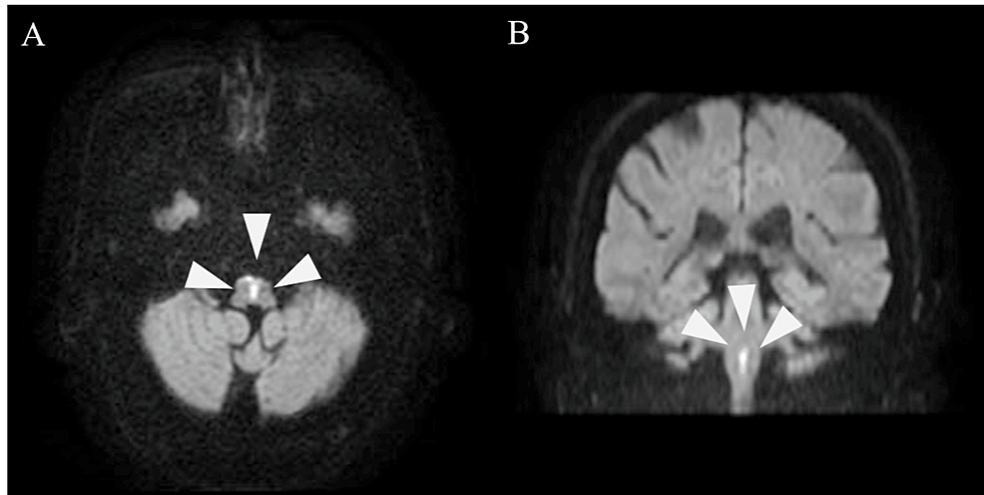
Head MRI findings on the day after admission. A: Axial DWI view. B: Coronal DWI view. High signal in the bilateral medial medullary in both A and B. MRI: magnetic resonance imaging; DWI: diffusion-weighted imaging
